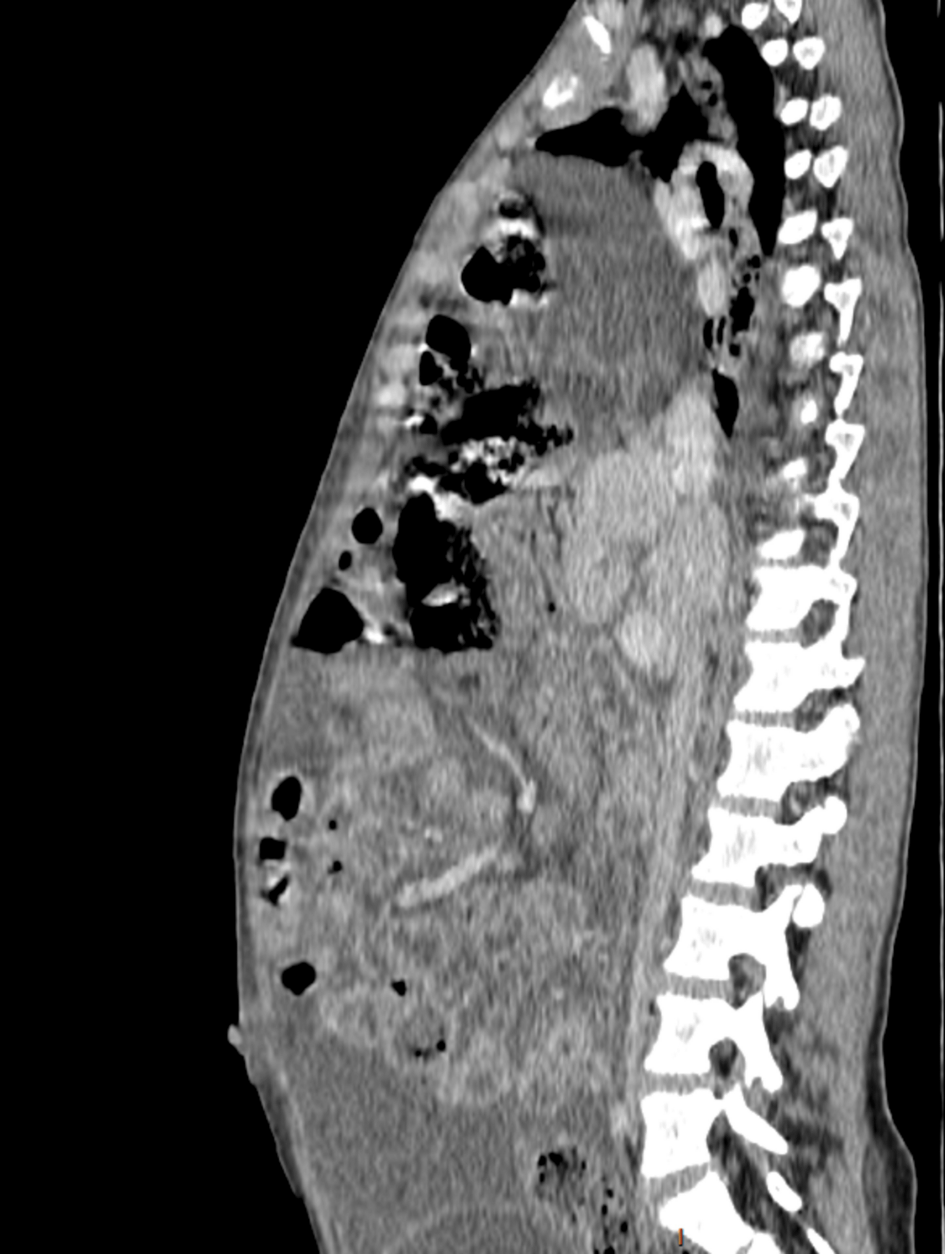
Sagittal contrast-enhanced CT of the chest and abdomen showing herniation of abdominal viscera into the thoracic cavity through the diaphragm CT: computed tomography
